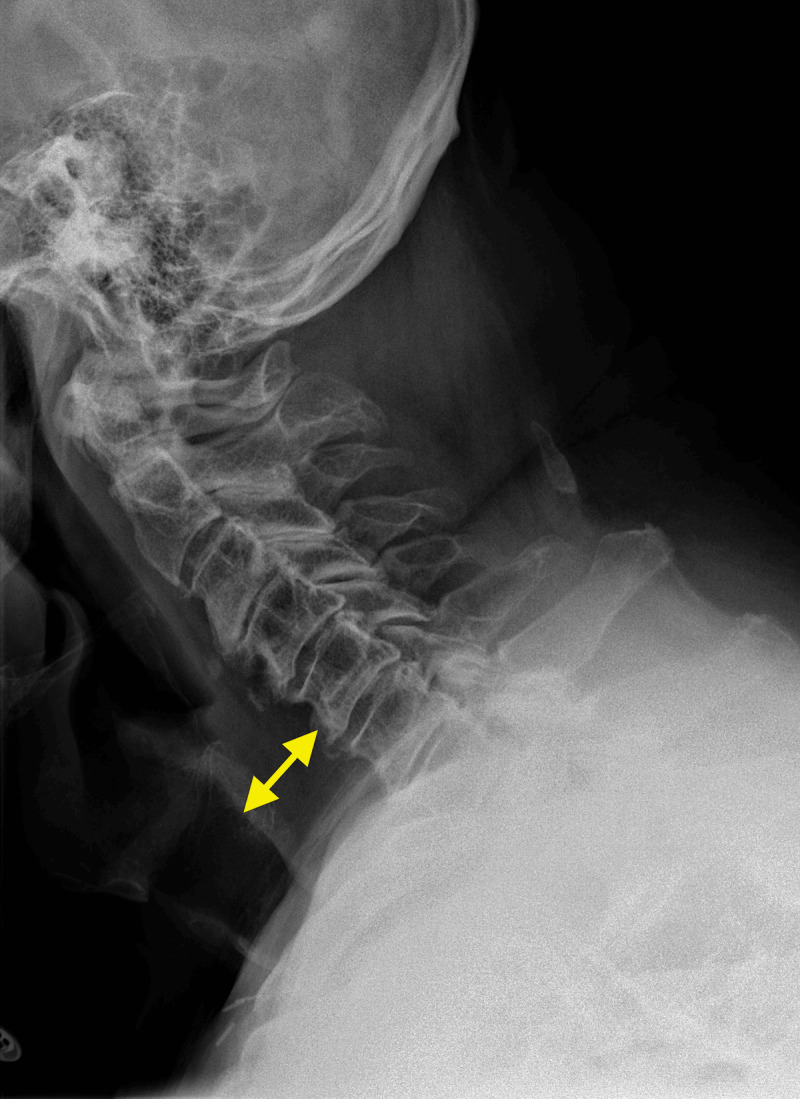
Lateral cervical X-ray showing soft tissue thickness of 30 mm in front of C5 (double arrow).
